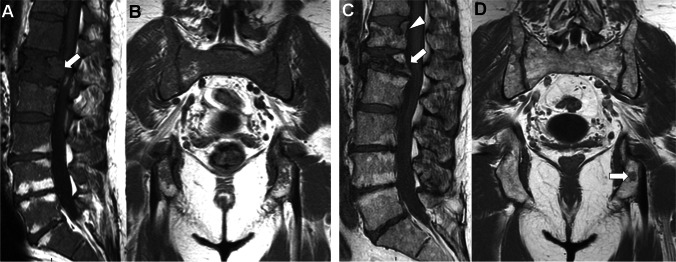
52 year-old patient with newly diagnosed MM (IgA lambda, stage IIIA, ISS 3): initial and post-ASCT axial skeleton MRI findings. A, B Baseline T1- weighted sagittal MR image of the lumbar spine (A) and coronal image of the pelvis (B) performed for the workup of acute lumbar pain show abnormal diffuse low signal intensity of the bone marrow, with pathologic fracture of the L1 vertebral body with mass effect on the spinal canal (arrow in A), leading to the diagnosis of MM. C, D Corresponding MR images obtained after high dose cytotoxic treatment and ASCT show return to normal signal intensity of the bone marrow, decrease in the mass effect of the L1 vertebral compression fracture (arrow in C). Note the appearance of a new vertebral fracture involving the upper endplate of the T12 vertebral body (arrowhead in C), and right ischiatic focal lesion suggesting residual abnormalities (arrow in D)
